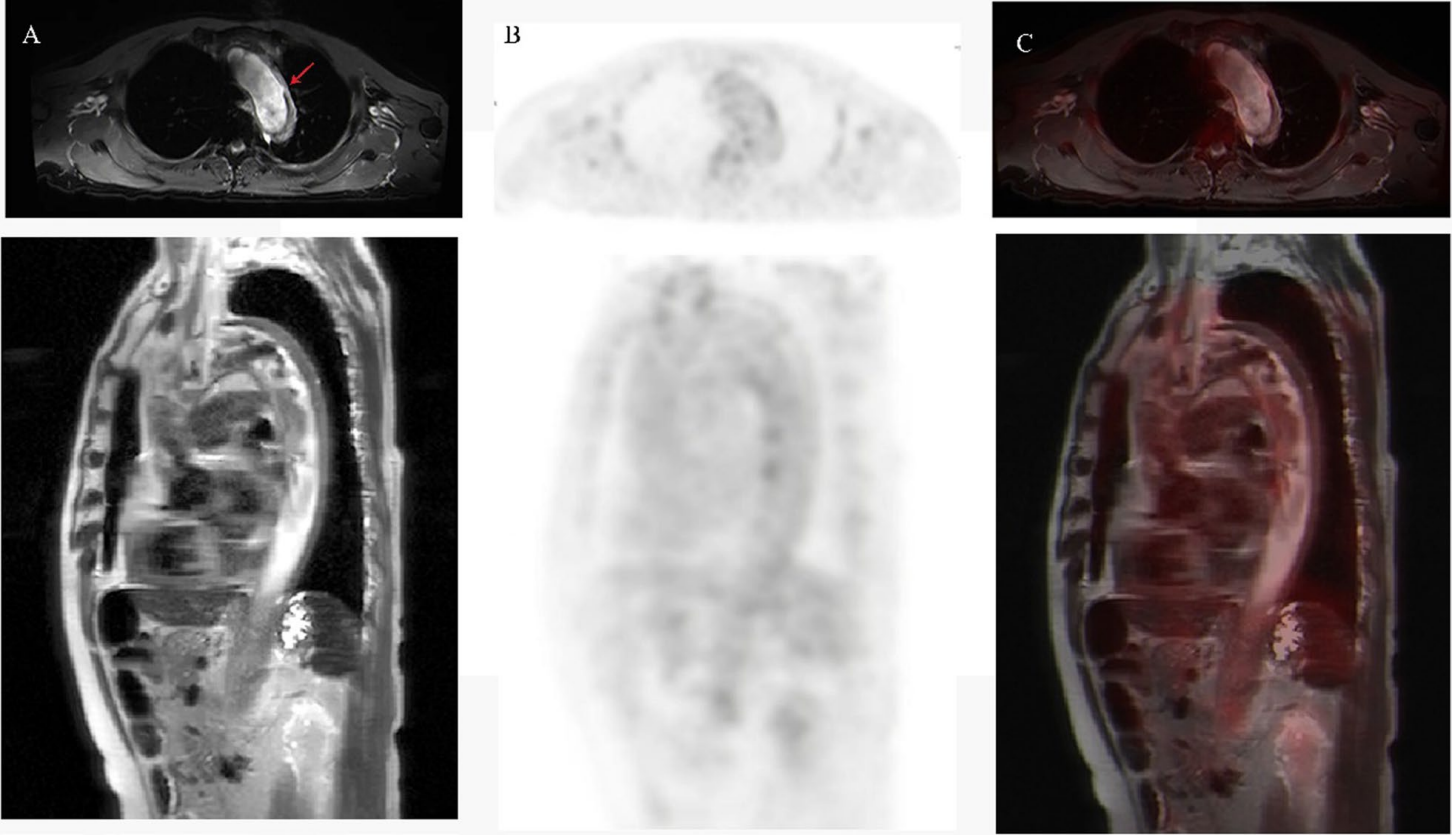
^18^F-fluorodeoxyglucose positron emission tomography/ magnetic resonance imaging. A Magnetic resonance imaging shows intramural hematoma with newly developed ULP on the arch (arrow). B The metabolism of the aortic wall was assessed by ^18^F-fluorodeoxyglucose positron emission tomography. C Fusion image of intramural hematoma
